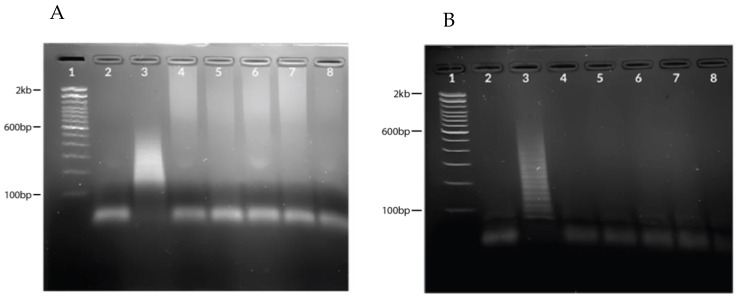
Gel image showing evaluation of clinical samples. (A) Hybridization chain reaction results using SARS-CoV-2 positive samples. Lane 1: 100 bp ladder, Lane 2: NTC, Lane 3: target, Lanes 4–8: positive samples (CT = 23.77, 27.96, 29.29, 31.07, 32.64, respectively). (B) Hybridization chain reaction results using SARS-CoV-2 negative samples. Lane 1: 100 bp ladder, Lane 2: NTC, Lane 3: target (positive control), Lanes 4–8: negative samples (CT = not detected for all samples).
